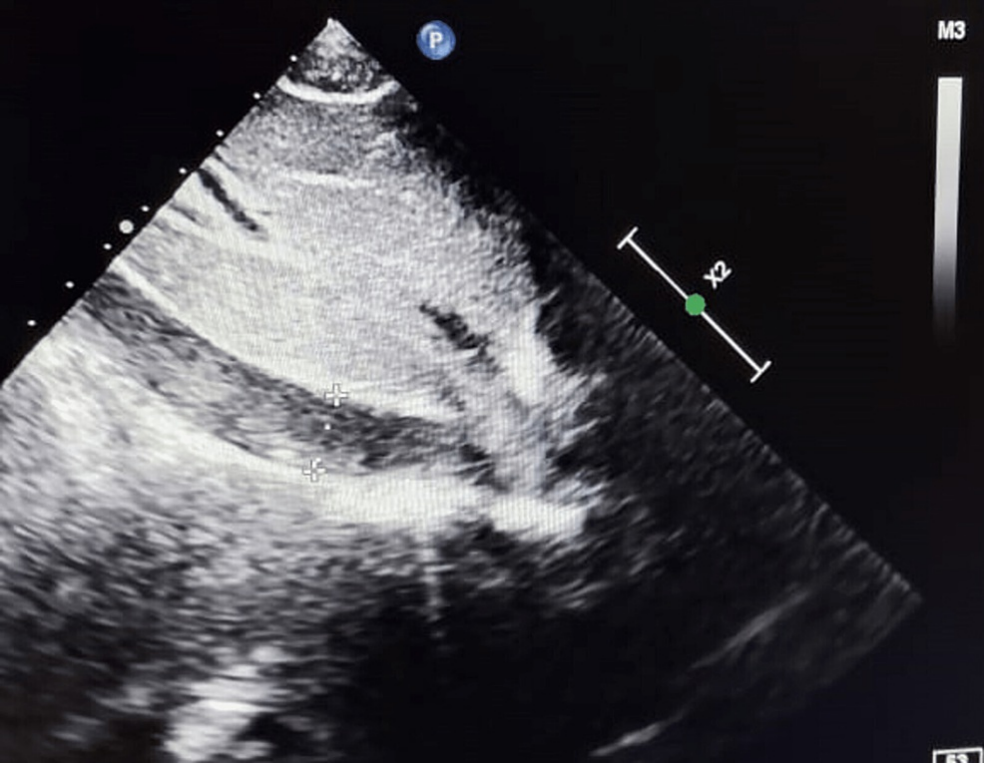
In the subcostal view of TTE, the inferior vena cava appears filled with thrombus TTE: transthoracic echocardiography
